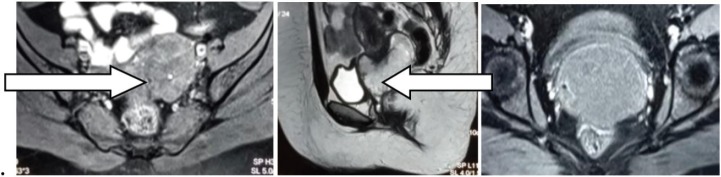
MRI of the pelvis; T2W and SPAIR hyper-intense polypoidal mass arising from the cervix measuring 6.2 × 6.9 × 7.2 cm^3^.
